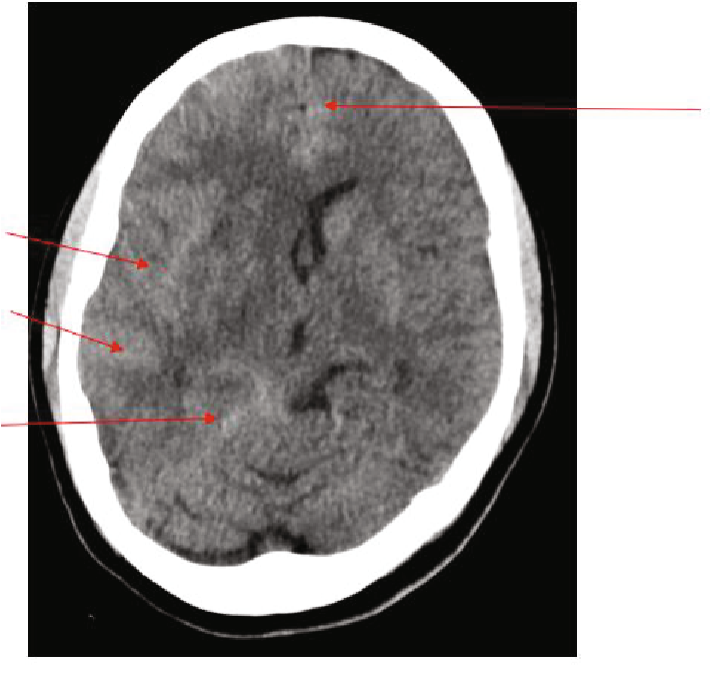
CT brain showing diffused subarachnoid blood with effaced cortical sulcal spaces.
